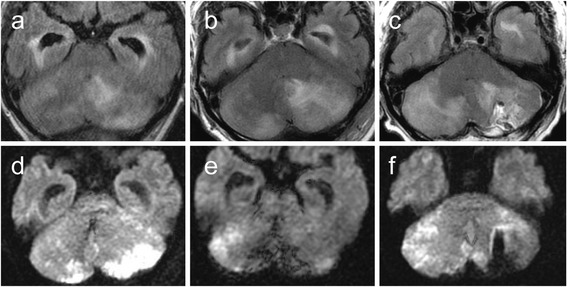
Progression of cerebellar infarction in Cryptococcal meningoencephalitis. a, d FLAIR (a) and DWI (d) performed following internal and external decompression 10 days after admission. The ischemic lesion progressed to the contralateral cerebellar hemisphere with peripheral edema and ventricular dilatation unresponsive to external continuous ventricular drainage. b, e. FLAIR (b) and DWI (e) performed 17 days after admission. The ischemic and edematous lesion progressed from dorsal to ventral. c, f FLAIR (c) and DWI (f) performed 23 days after admission, 3 days before the patient died. The cerebellum had completely swollen, and tight cisterns were observed indicating brain herniation
